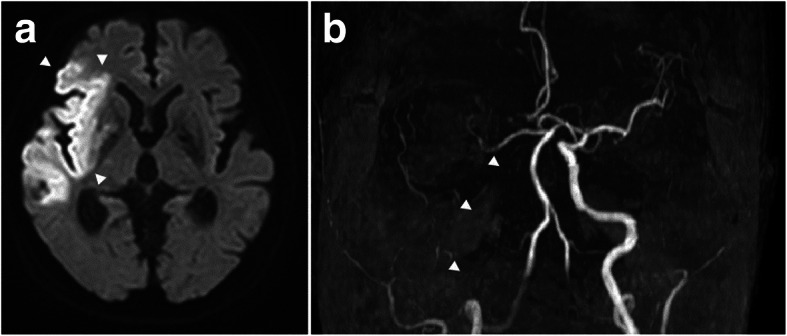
Magnetic resonance imaging (MRI) of case 1. a Diffusion weighted MRI revealing edema following a right lobe cerebral infarction (arrowheads). b Maximum intensity projection of MRI showing complete occlusion of the right internal carotid artery by a thrombus. Arrowheads indicate the right internal carotid artery with defect
